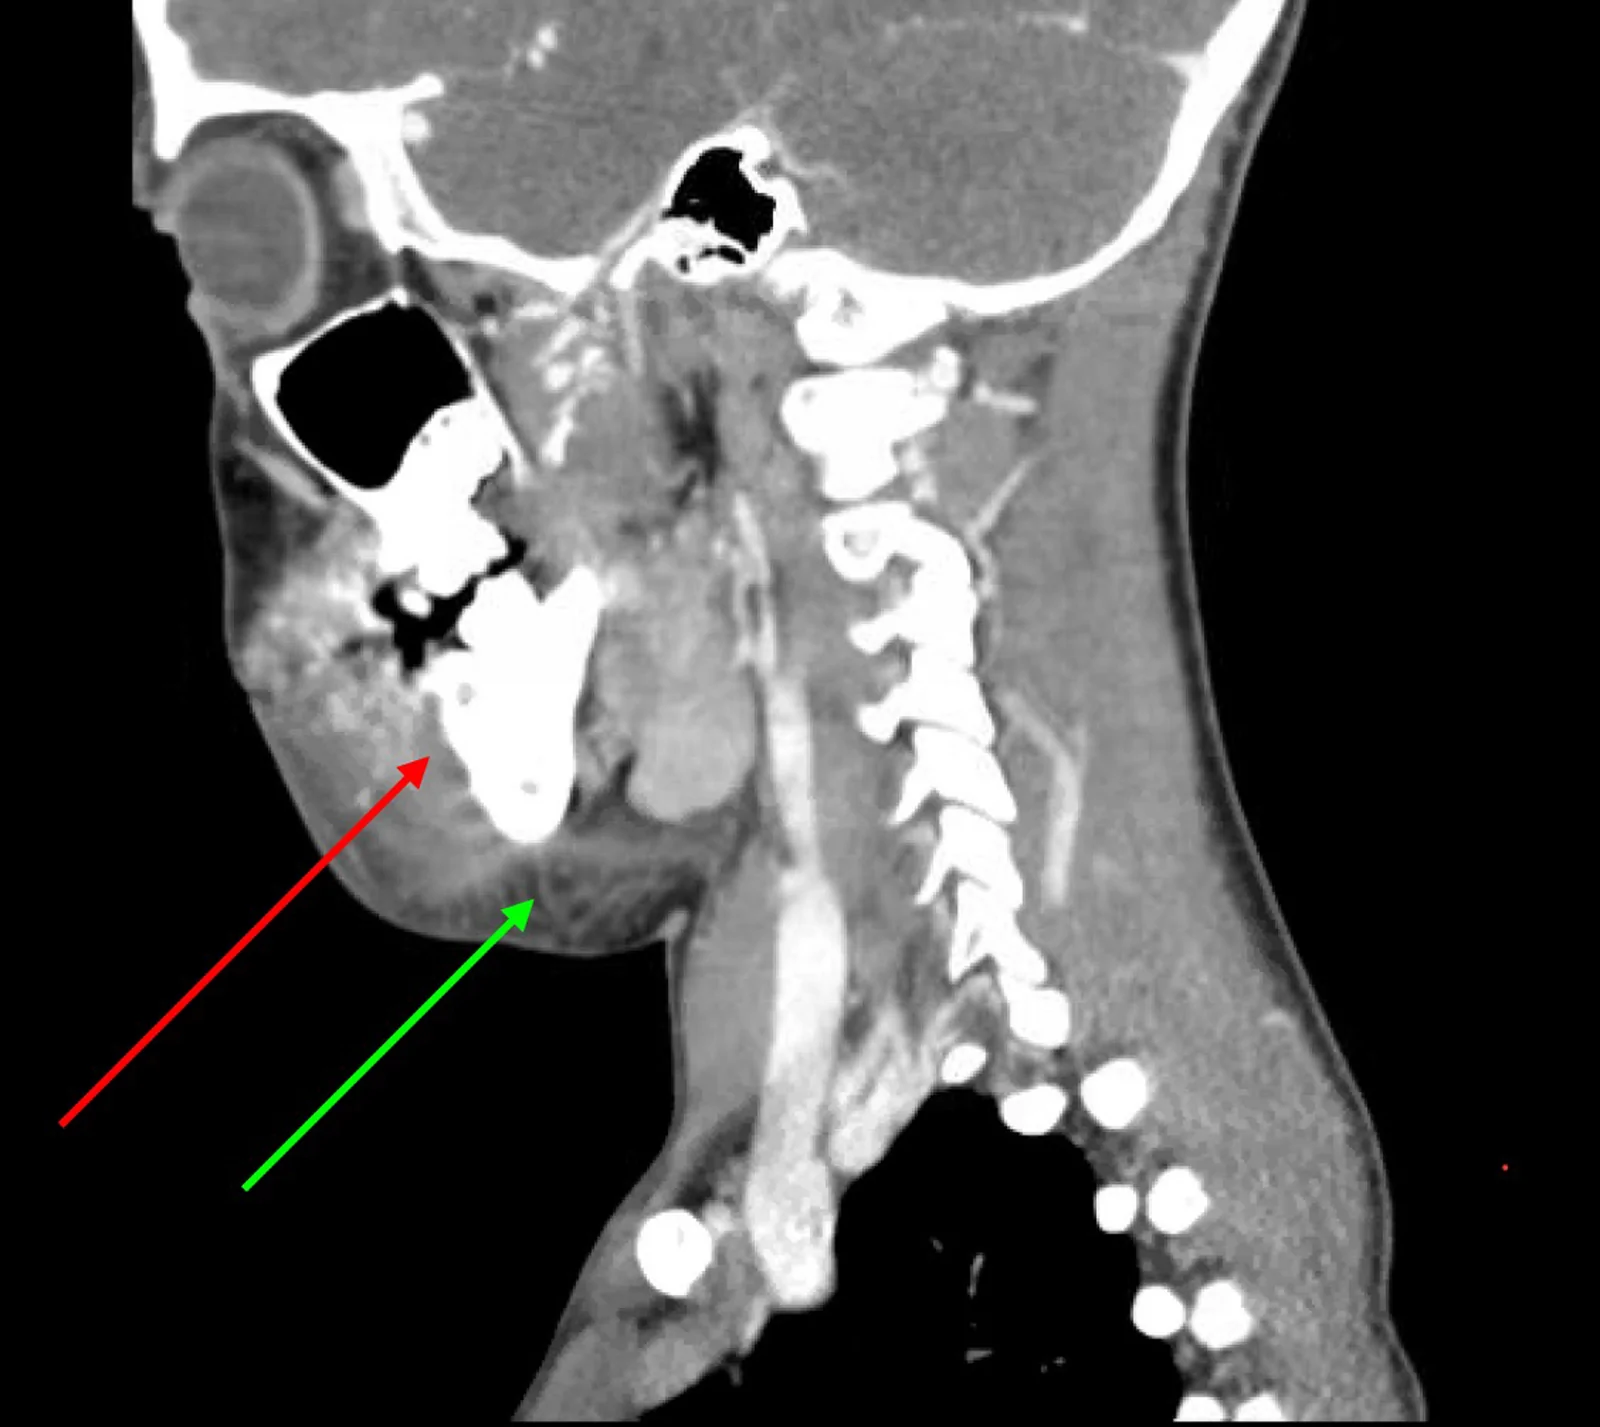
Sagittal view of CT scan—soft tissue of the neck. The red arrow denotes the subperiosteal abscess and the green arrow denotes the inferior part of the facial cellulitis. CT, computed tomography.
